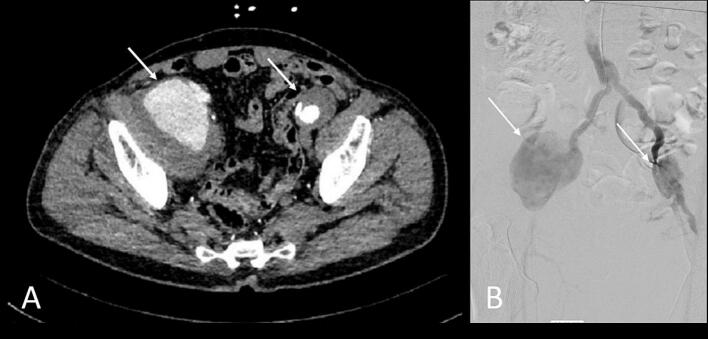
A) Preoperative abdominal CTA showing two distal para-anastomotic aneurysms, indicated by arrows, measuring 70 mm (extending to the right iliac artery) and 45 mm (extending to the left iliac artery) in maximal diameter (case 2), B) the two pseudoaneurysms shown in preoperative digital subtraction angiography.
